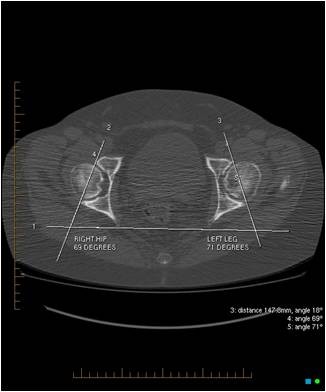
The acetabular version calculated by plotting a trans-ischial line across the ischial tuberosities on axial CT image. A second line is drawn across the anterior and posterior margins of the bony acetabulum. The anteversion angle is formed by the intersection of these two lines.
